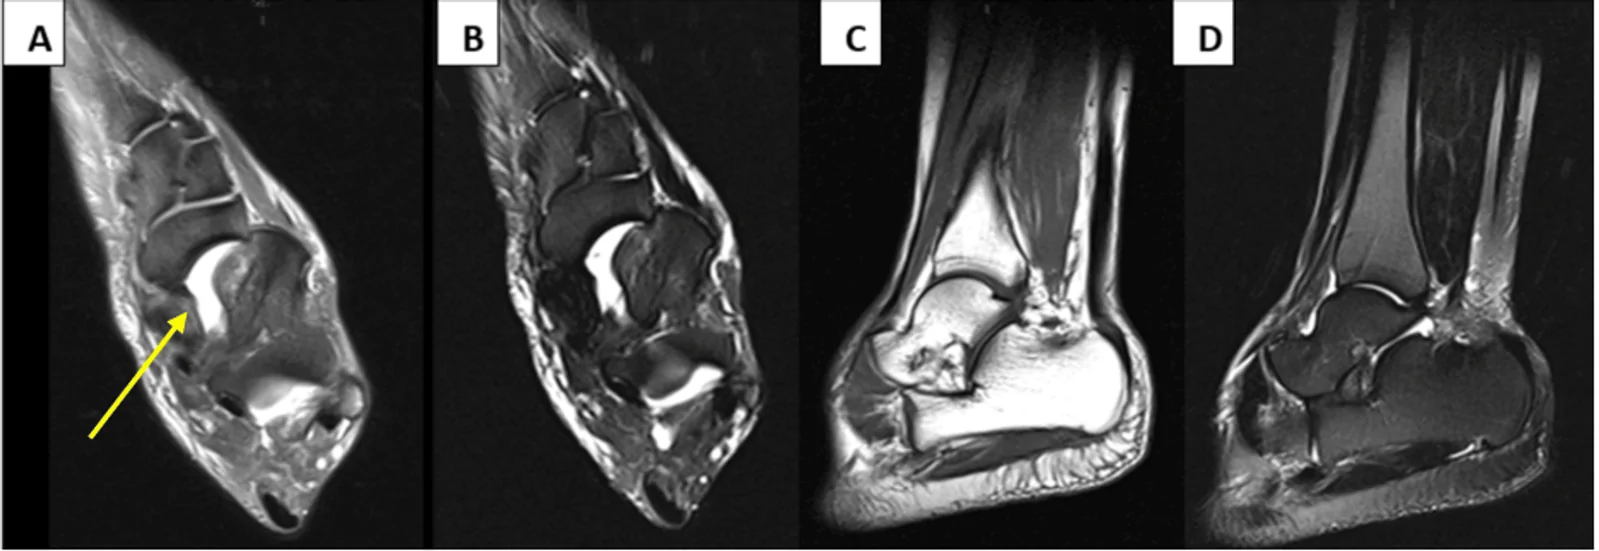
Preoperative MRI images (A-D) 30 days after injury showing left foot medial swivel injury Arrow showing medial talonavicular dislocation MRI: magnetic resonance imaging
